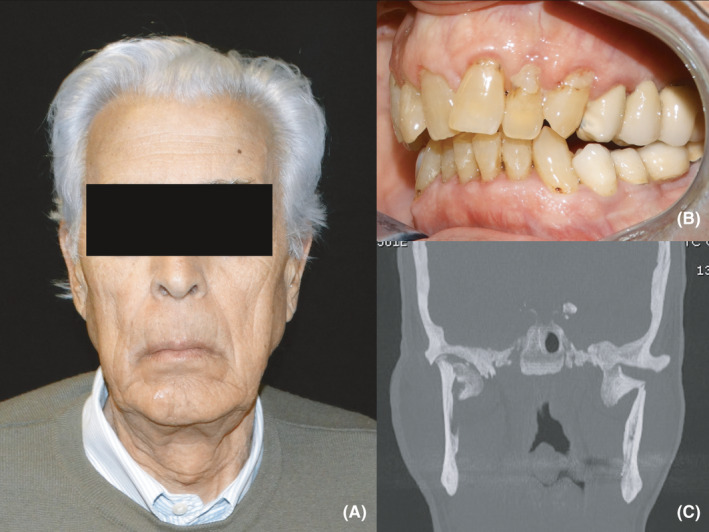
The patient at the first clinical examination. (A) Frontal view. (B) Intraoral photograph shows dentulous upper and lower arches, increased overjet and mild anterior open bite. (C) Coronal CT scan demonstrates bilateral condylar fractures
